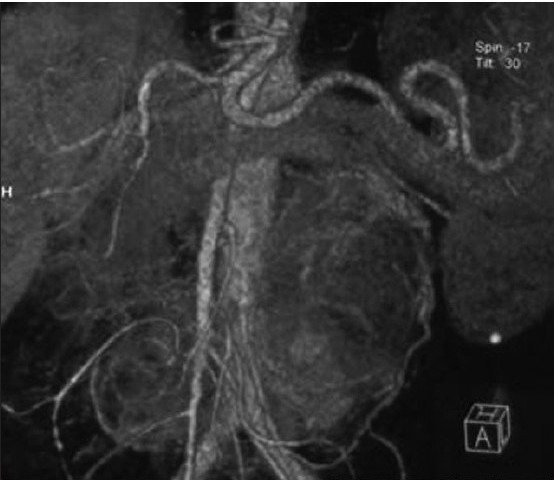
MSCT shows a round or similarly round retroperitoneal tumor with inner central hemorrhage and necrosis. Tumors show obvious intensification after injection of constrast medium. a) Multiple extra-adrenal retroperitoneal paragangliomas. Coronal contrastenhanced reformatted CT scan shows two lobulated soft-tissueattenuation masses (arrows) above the aortic bifurcation
